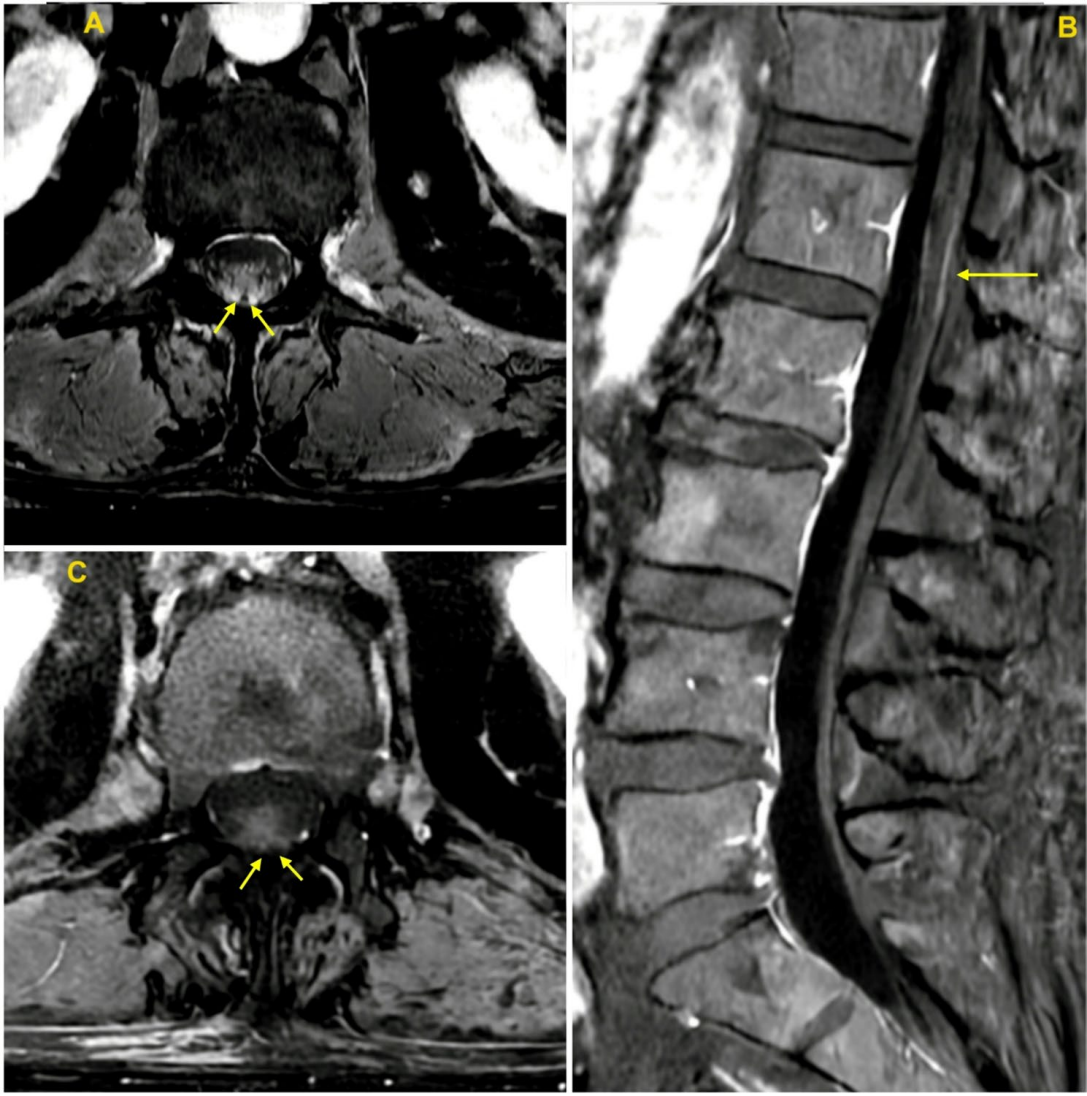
Initial and delayed spinal imaging (A) Axial and (B)Sagittal T1 post-gadolinium MRI obtained on day 5 of illness showing thickening and avid enhancement of the dorsal cauda equina roots (yellow arrows) at the level of the conus medullaris. (C) Axial T1 post- gadolinium MRI obtained 3 months after illness showing persistent, though markedly decreased, cauda equina root thickening and enhancement (yellow arrows)
